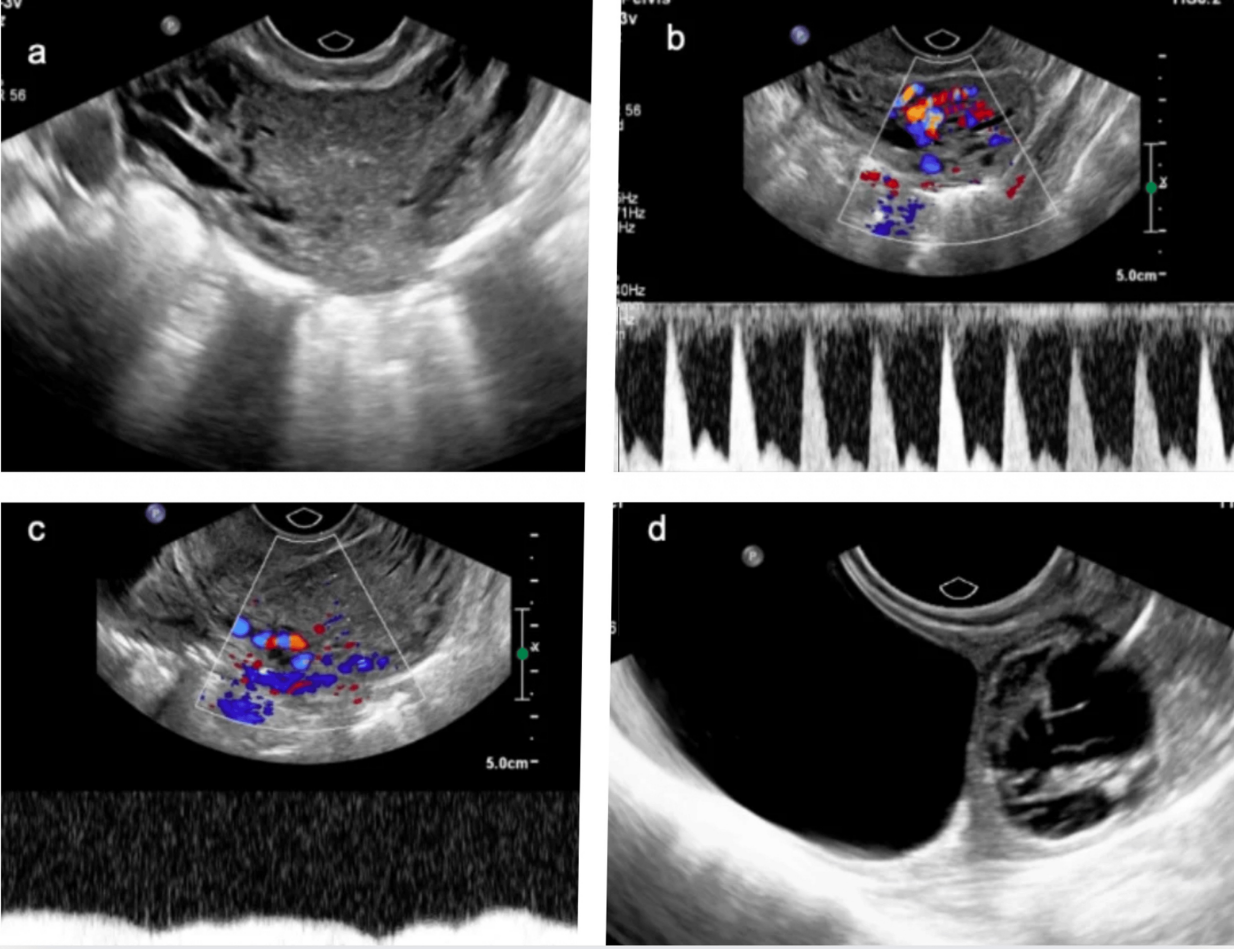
Ultrasound transvaginal sonography grayscale, color Doppler, and spectral Doppler images. a: Bulky uterus with serpiginous vessels in the myometrium and parametrium. b, c: Color Doppler study showing arterial and venous waveforms. d: Simple ovarian cyst and hemorrhagic ovarian cyst in the left ovary.
